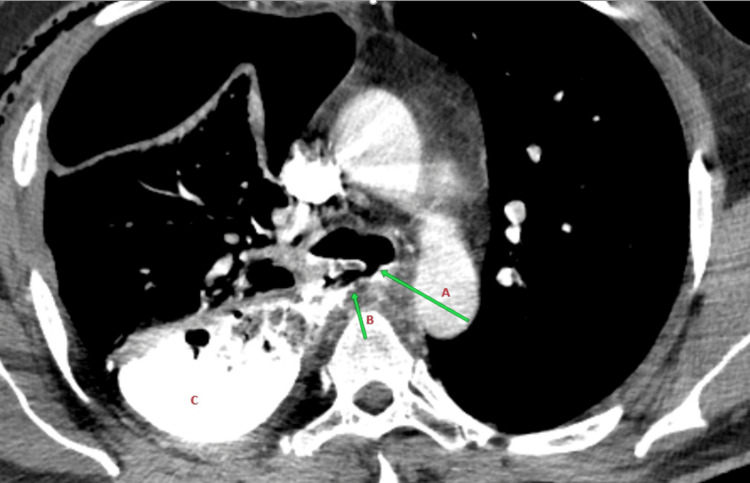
Computed tomography (CT) with contrast after tracheal perforation (A) Tracheal Perforation just above Carina (B) Oesophageal Perforation (C) Contrast Pooling in the Right Pleural Cavity
